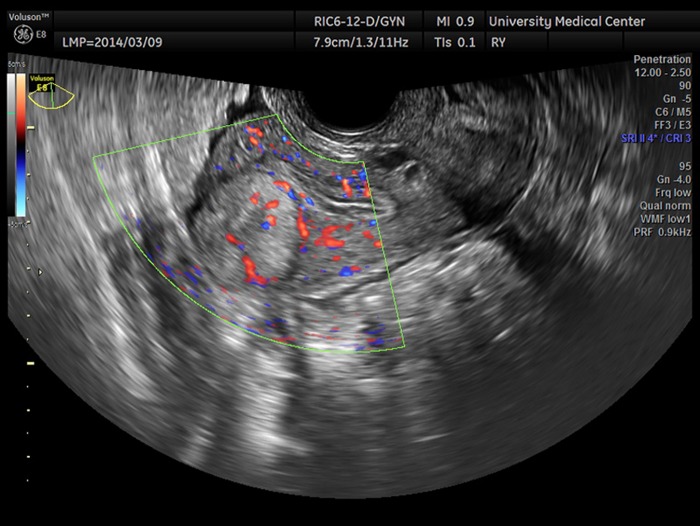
Transverse transvaginal ultrasound image of the upper aspect of the uterus obtained on March 17, 2014, showing a 2.74 cm mostly echogenic mass with a hypoechoic rim extending from the serosa to the endometrium.
